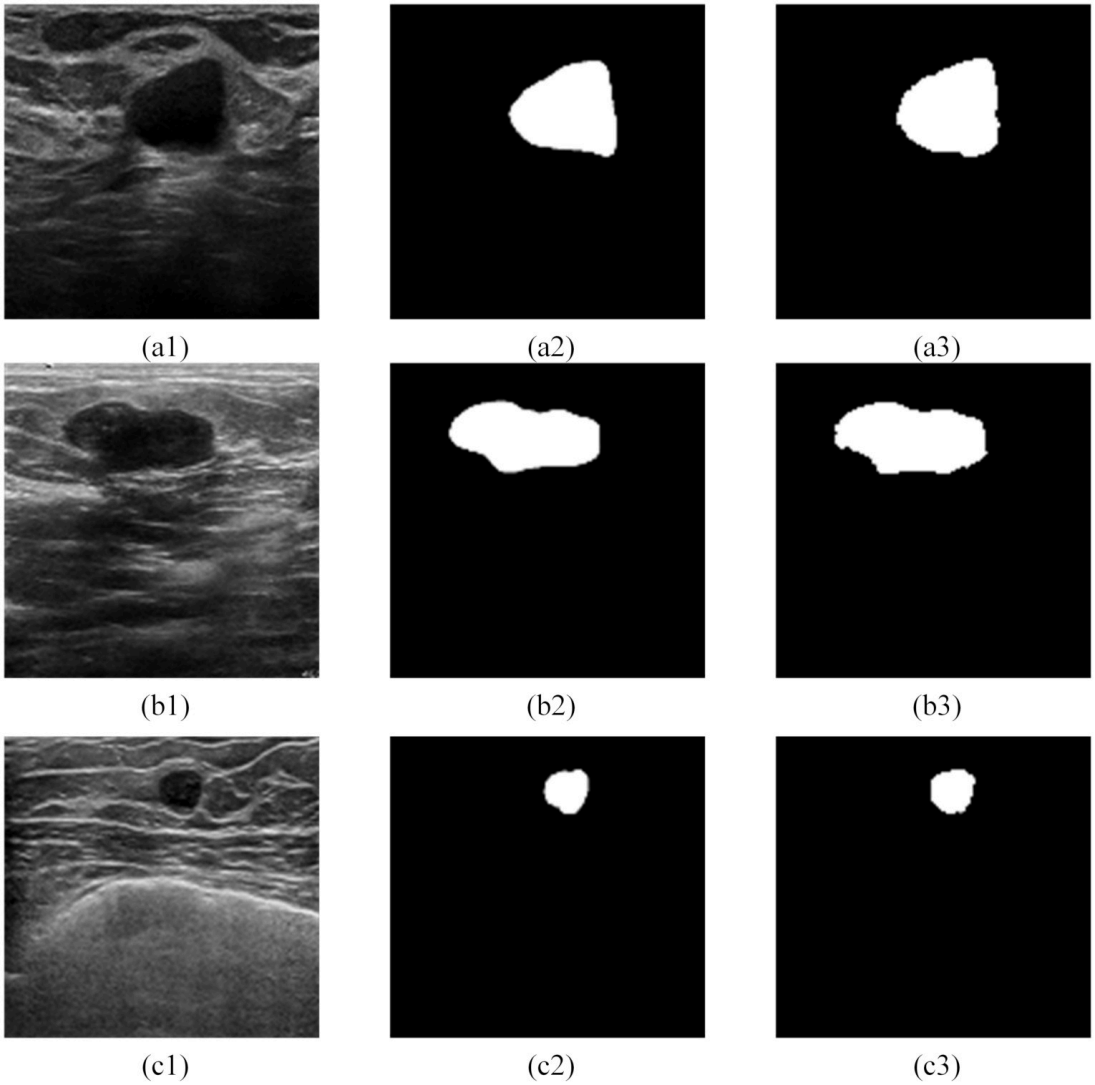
Ultrasound breast tumor segmentation based on the RDAU-NET model. Here (a1)—(c1) were obtained from Dataset B [13]. (a2), (b2), (c2) are gold standard and (a3), (b3), (c3) are the results of the RDAU-NET model segmentation.
